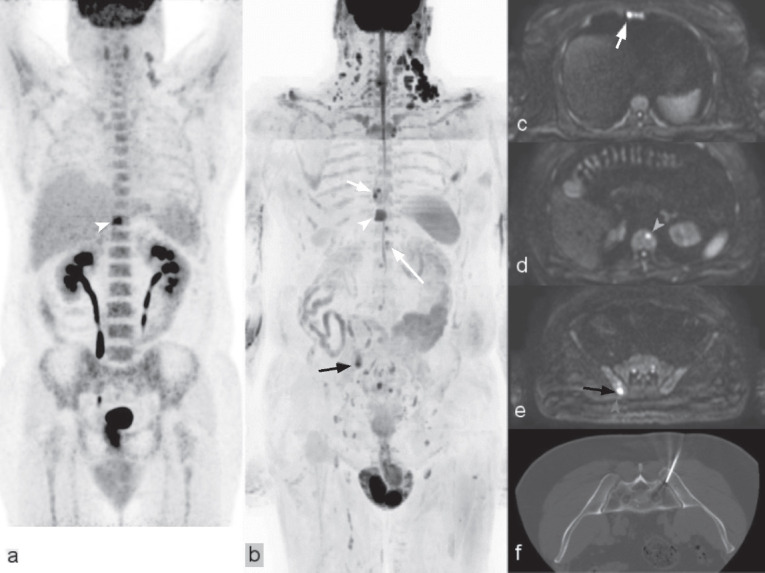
Bone lesions in lymphoma. A 51-year-old male with follicular lymphoma (G1-G2). (a): ^18^FDG-PET for distant staging to have a single bone metastasis of the T10 vertebra (white arrowhead). At WB-MRI 1 month later, oligometastatic disease with multiple bone lesions was evident in (b): the inverted grey scale MIP of the b-900 diffusion weighted images; with corresponding hyperintensities being seen in the axial source images (c–e). In addition to the T10 metastasis (white arrowhead in a and b), a sternal metastasis (smaller white arrow in b and c), a second thoracic vertebral lesion (longer white arrow in b and d) and an iliac lesion (black arrow in b and e). Metastasis in the right iliac bone was confirmed by CT-guided biopsy (f).
